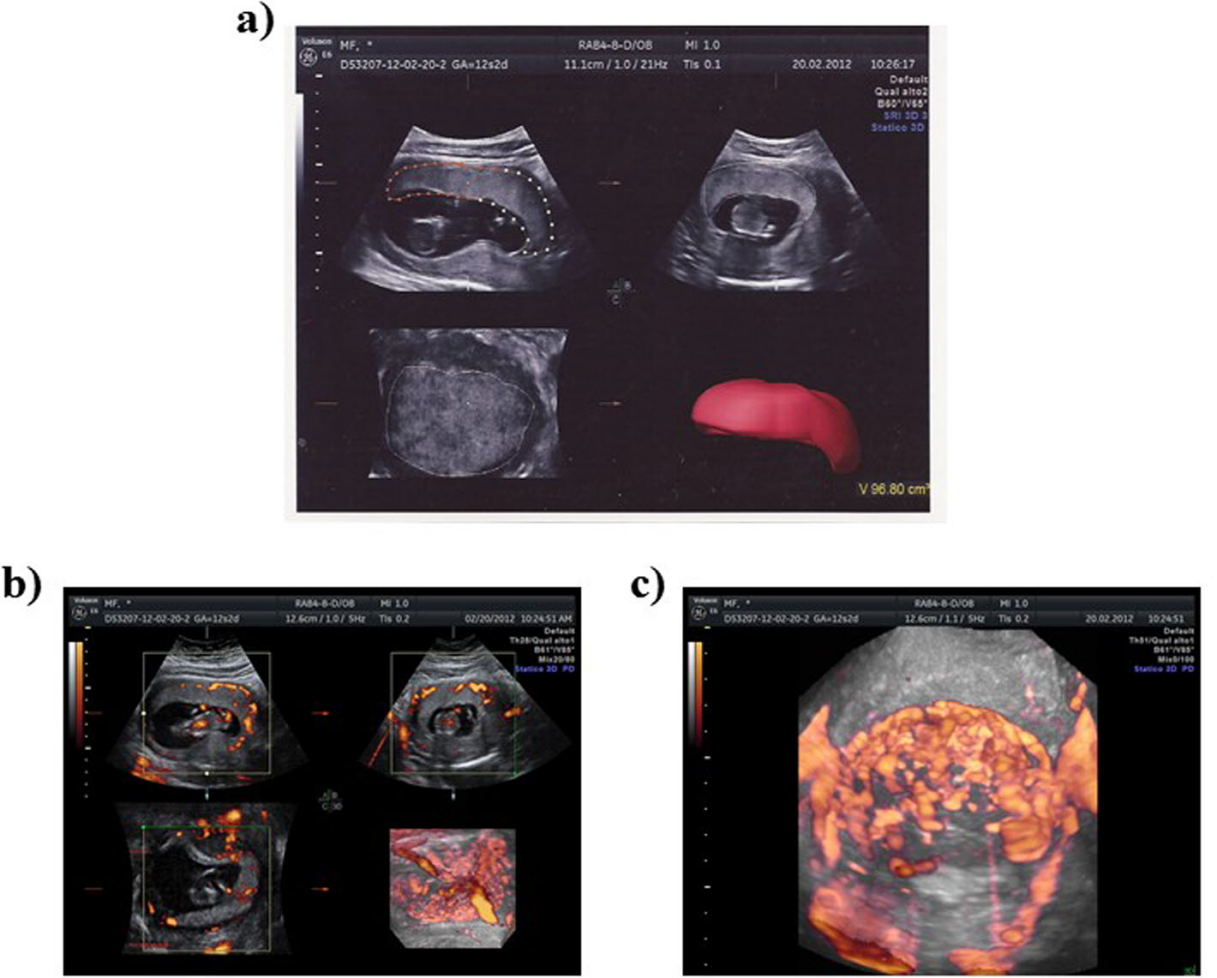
3D power Doppler acquisition images. a 3D power Doppler image of a placenta at 12 + 2 weeks in a multiplanar mode. Placenta rotated horizontally. Image shows the placental bed after tracing. b Sampling of placental volumes by 3D sonography: Two dimensional ultrasound and Power Doppler imaging of the placental vessels. c: 3D volume rendering
